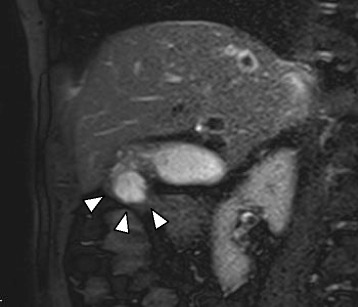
T2-weighted magnetic resonance imaging demonstrated a cystic mass at the gallbladder fundus (*white triangles*)
